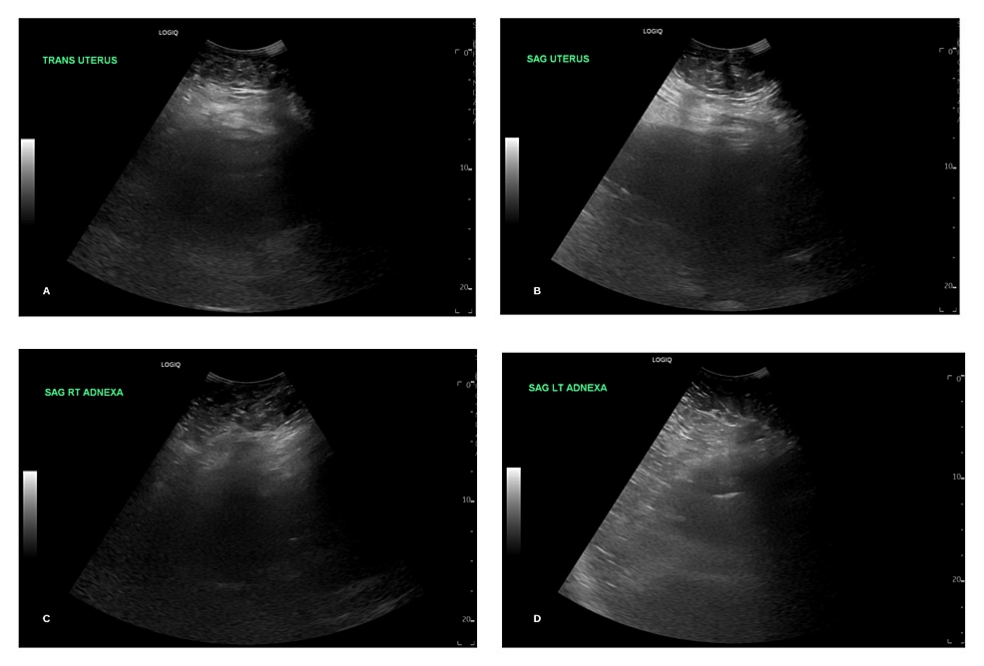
Pelvic ultrasound demonstrating negative findings; A: Transverse view of the normal uterus; B: Sagittal view of the normal uterus; C: Sagittal view of the normal right adnexa; D: Sagittal view of the normal left adnexa.
